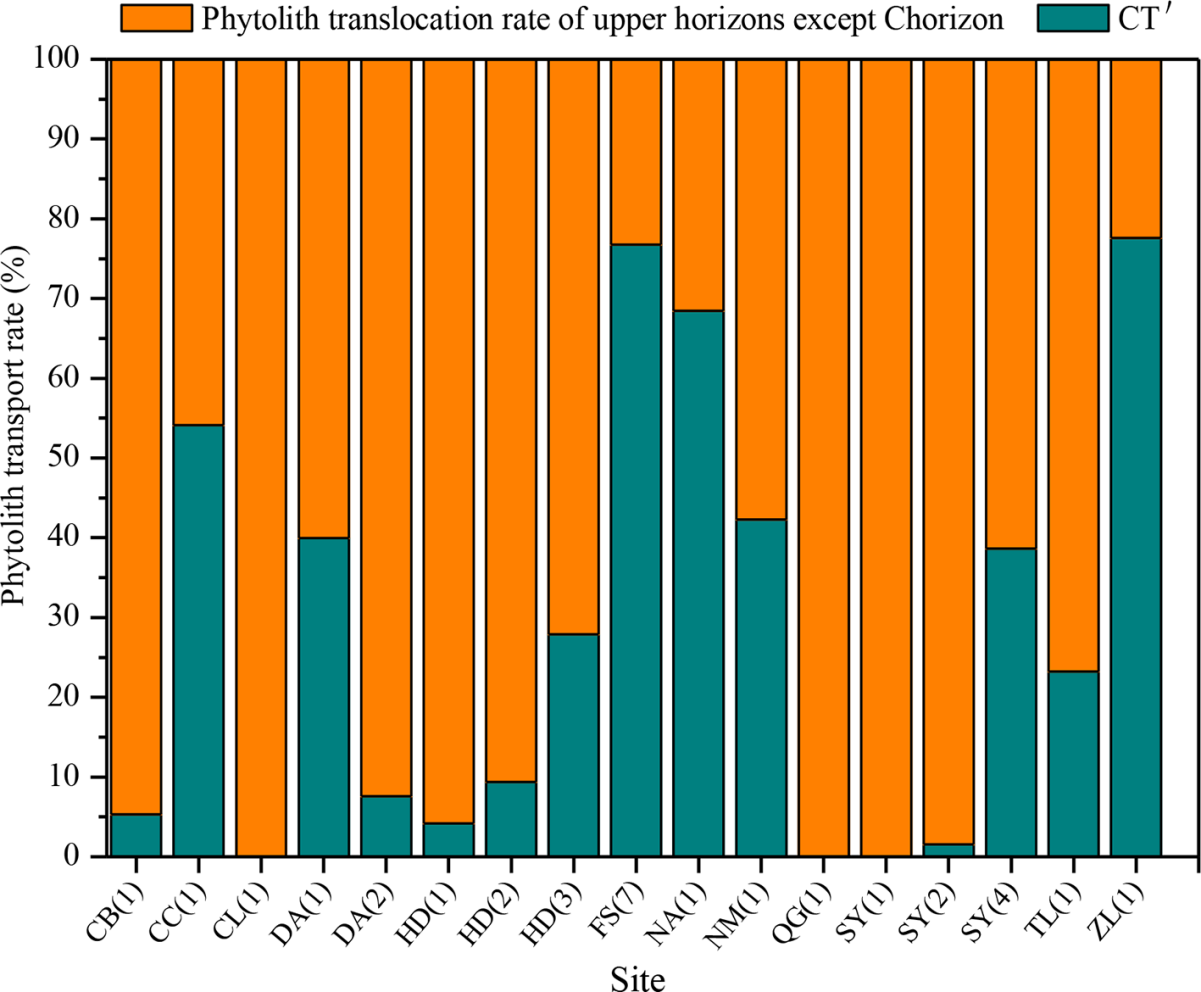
Relative translocation rate of phytoliths to the C horizon of (*CT*′) of soils in Northeast China.
